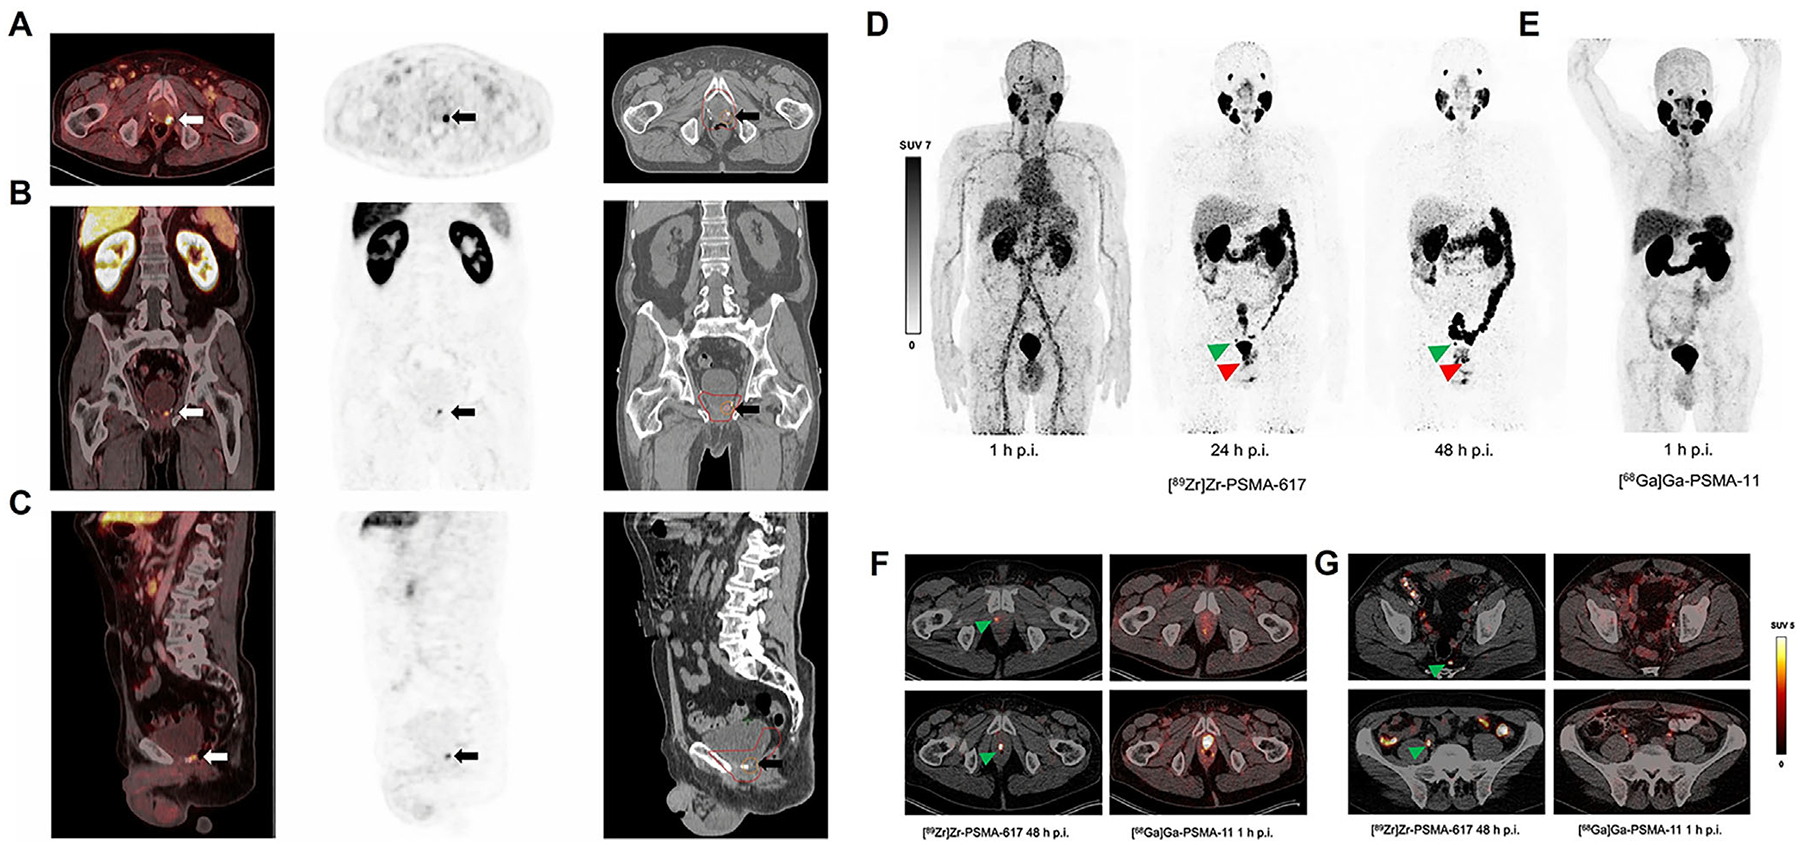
(A–C) [^18^F]-flotufolastat positron emission tomography (PET)/computed tomography (CT)-guided salvage radiotherapy for local prostate cancer recurrence caudal to the bladder. Axial (A), coronal (B), and sagittal (C) PET/CT fusion images show focal uptake (arrows) with corresponding radiotherapy planning contours: red = planning target volume, orange = simultaneous-integrated boost, pink = gross tumor volume. Ref. 93 with permission from the Springer Nature, copyright 2025. (D, E) Maximum-intensity projection PET/CT images of a patient with biochemical recurrence of prostate cancer (PSA 2.5 ng/mL, PSA doubling time >12 months). (D) [^89^Zr]Zr-PSMA-617 PET/CT at 1, 24, and 48 h postinjection shows focal uptake indicating a local recurrence (red arrow) and a pelvic lymph node metastasis (green arrow). (E) [^68^Ga]Ga-PSMA-11 PET/CT at 1 h postinjection fails to reveal these lesions, highlighting the superior detection sensitivity of [^89^Zr]Zr-PSMA-617 for delayed imaging. (F) Transversal PET/CT images of two patients with presumed local prostate cancer recurrence. [^89^Zr]Zr-PSMA-617 PET/CT at 48 h postinjection (left column) clearly identifies focal uptake at the recurrence site (green arrows), whereas [^68^Ga]Ga-PSMA-11 PET/CT at 1 h postinjection (right column) shows no corresponding lesion. (G) Transversal PET/CT images of two patients with suspected pelvic lymph node metastases. [^89^Zr]Zr-PSMA-617 PET/CT at 48 h postinjection (left column) reveals metastatic lymph nodes (green arrows) that are not detected by [^68^Ga]Ga-PSMA-11 PET/CT at 1 h postinjection (right column), demonstrating the enhanced sensitivity of delayed [^89^Zr]Zr-PSMA-617 imaging. Ref. 93 with permission from the Springer Nature, copyright 2023.
